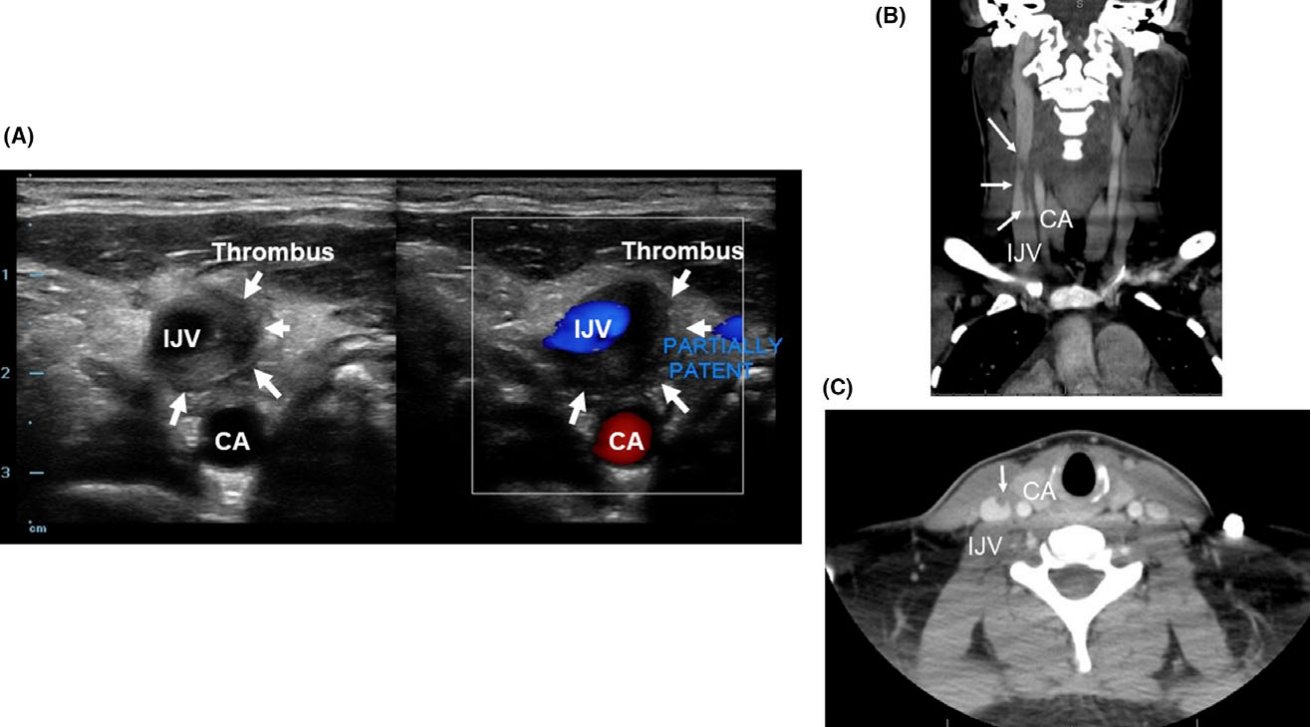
Venous duplex ultrasound (A) of a 37‐year‐old woman revealed acute thrombus (arrows) in right internal jugular vein (IJV) with partial patency. The coronal view (B) and axial view (C) on computed tomography scans of the neck revealed a thrombus within the right IJV at the level of the thyroid extending cranially (arrows). CA, carotid artery.
